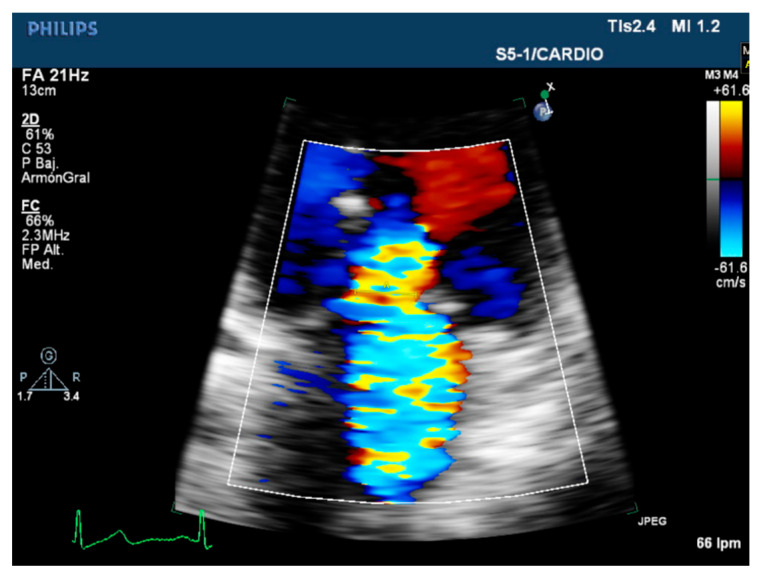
Transthoracic echocardiography. Vena contracta in severe mitral regurgitation.
